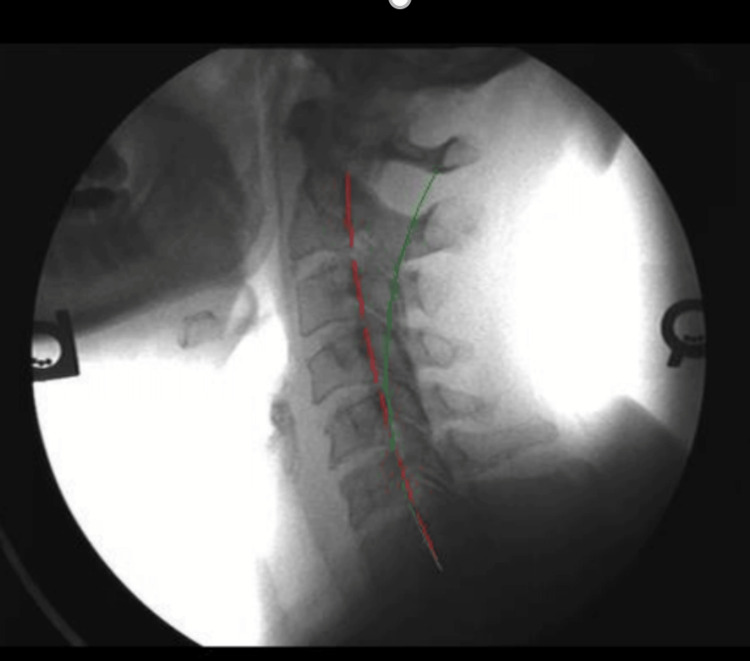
DMX image - 1 Lateral view with red dotted line showing the patient's curvature in a neutral position along the posterior longitudinal ligaments. The green line represents the normal spine position and normal curvature in a neutral position DMX: digital motion X-ray
